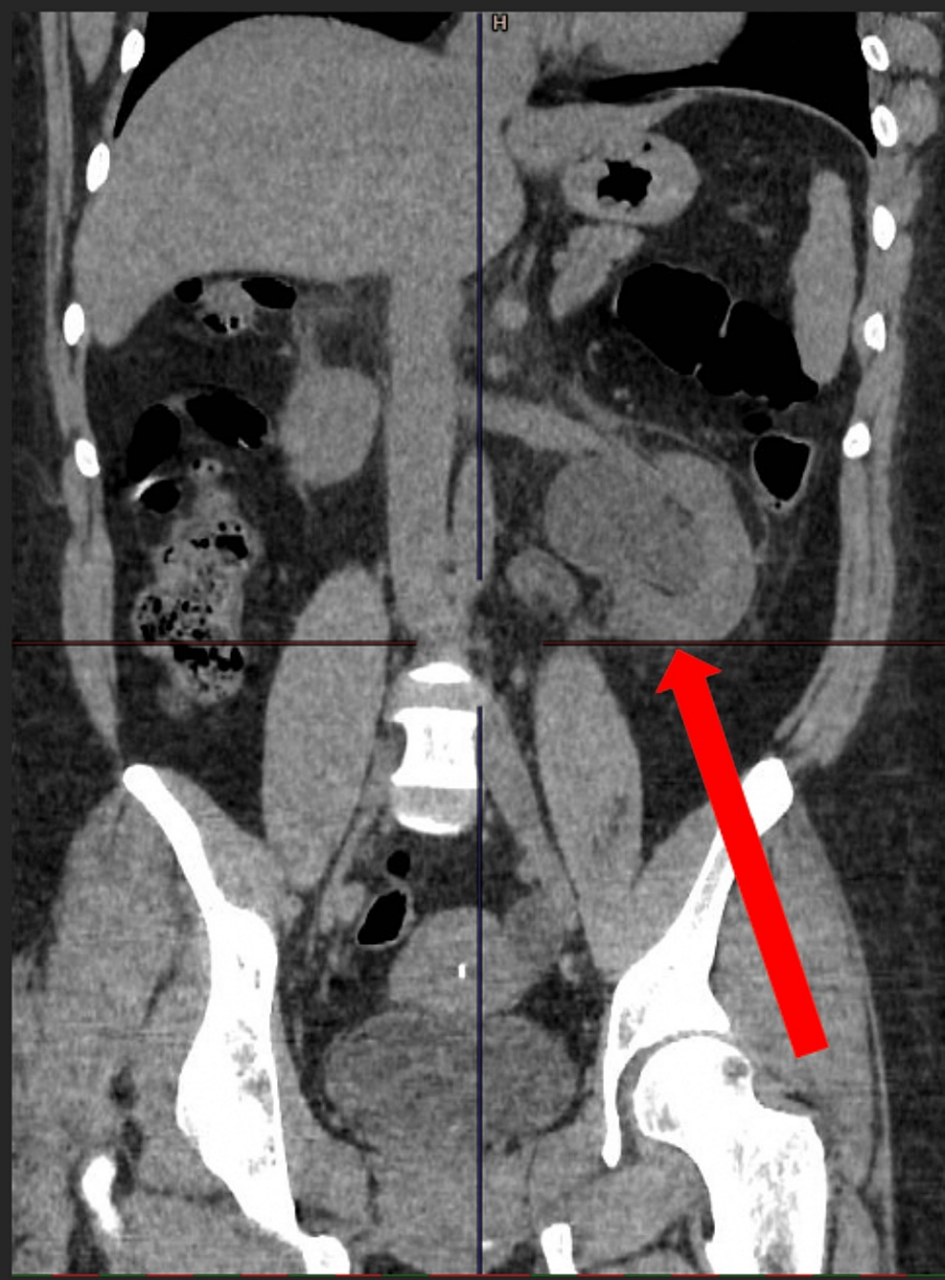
Unenhanced computed tomography scan of the kidneys, ureters, and bladder demonstrating left ureterohydronephrosis
